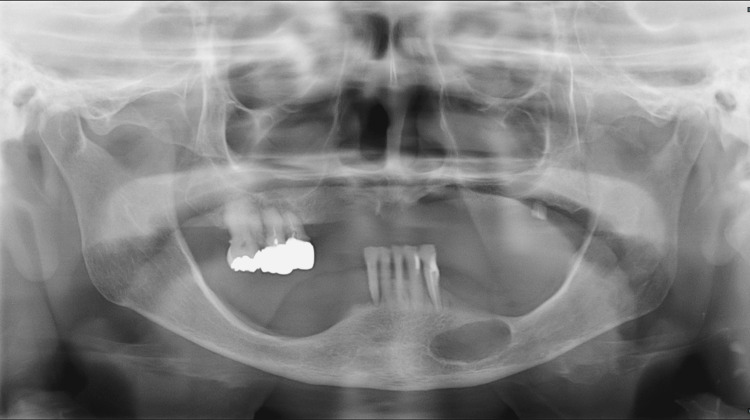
Panoramic X-ray on the initial visit. A clearly defined, internally homogeneous radiolucent area is observed from the mandibular region to the anterior area.
